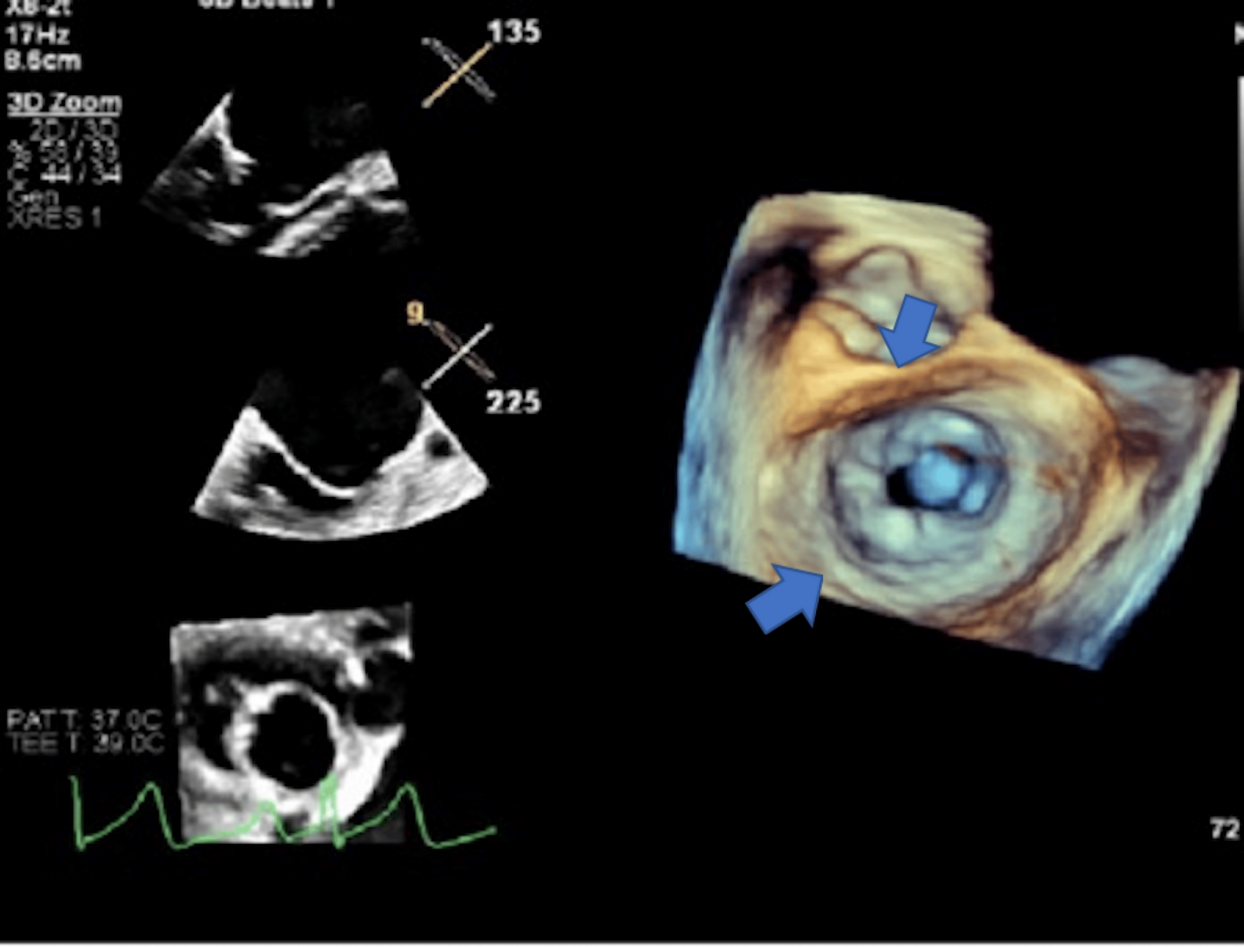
Transesophageal echocardiogram post mitral balloon valvuloplasty showing improvement in mitral valve area (blue arrows) to 1.9 cm².
